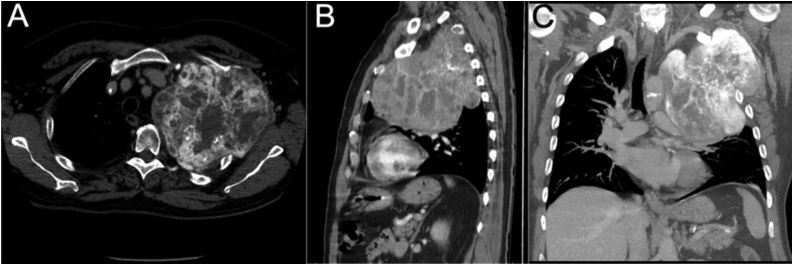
Initial pre-therapy CT images of the thorax in A) axial, B) sagittal, and C) coronal views.
